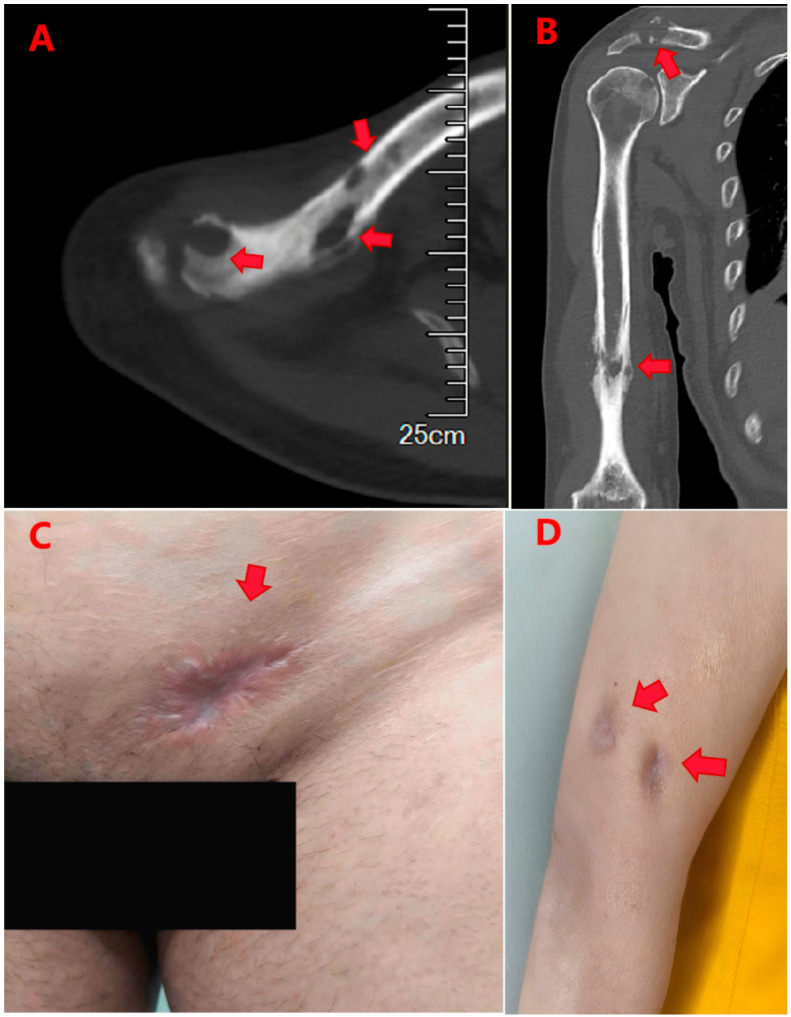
Disease progression and treatment response during follow-up. (A) Chest CT showing multiple osteolytic lesions in the right clavicle (red arrows). (B) Chest CT demonstrating osteolytic lesions in the right clavicle and distal humerus (red arrows). (C) Photograph of the left inguinal region showing complete wound healing following combination therapy (red arrow). (D) Photograph of the right upper extremity showing resolution of swelling and complete healing after continued treatment (red arrow).
